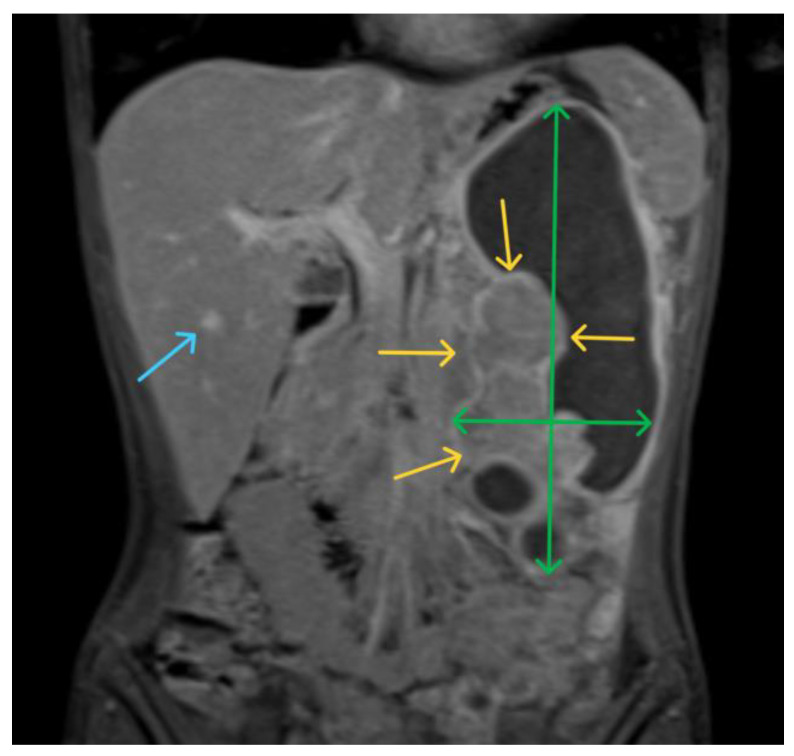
MRI T1 sequence of the abdomen, coronal section in the venous phase: Secondary hepatic lesion with gadolinium uptake, subcentimetric (blue arrow). Tumor mass (green arrows) with a solid component showing gadolinium uptake (yellow arrows) and a liquid component.
